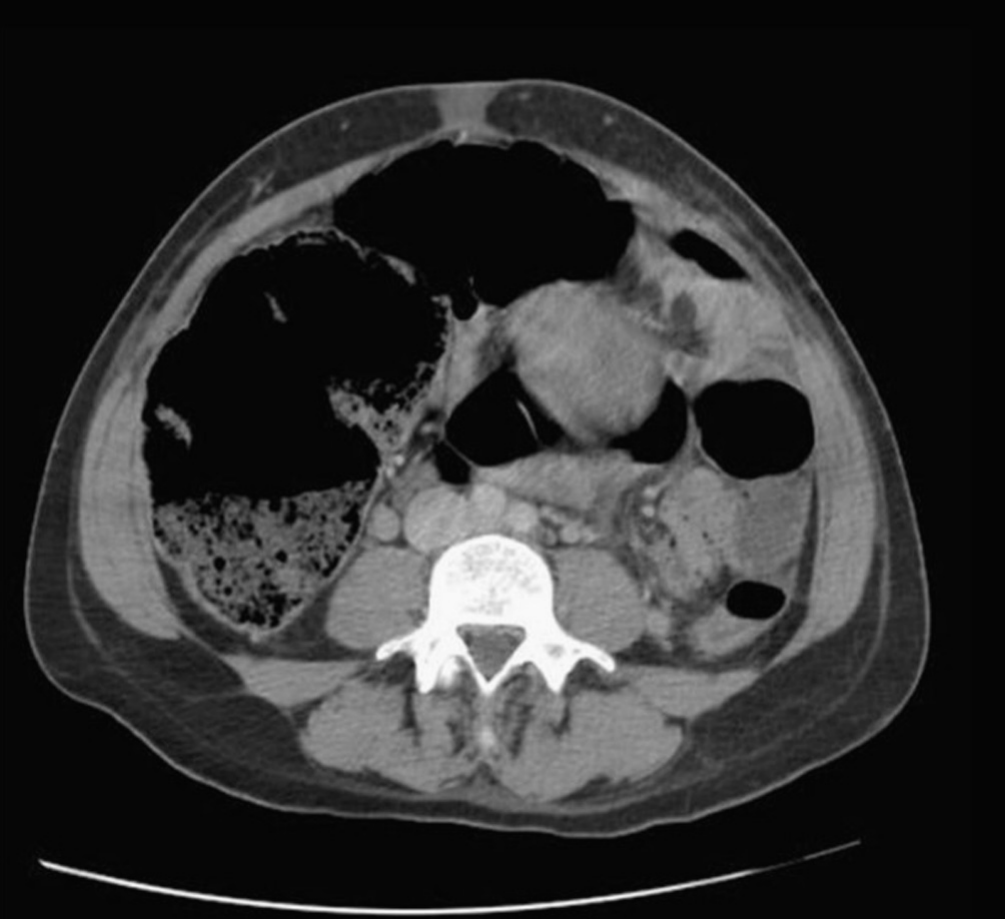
Abdominal computed tomography image showing marked caecal dilatation.
